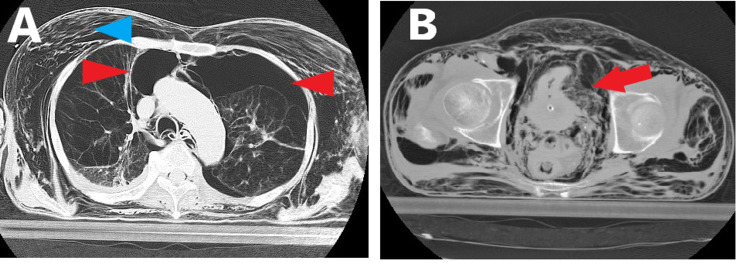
CT scan after the ROSC. CT showed massive retroperitoneal emphysema, subcutaneous emphysema (A, blue arrowhead), intra-abdominal air, and bilateral pneumothorax (A, red arrowhead). Although it was difficult to identify the site of injury due to intra-abdominal air and retroperitoneal emphysema, the diagnosis of bladder rupture was made due to the irregularity of the bladder wall (B, red arrow). CT: computer tomography; ROSC: return of spontaneous circulation
